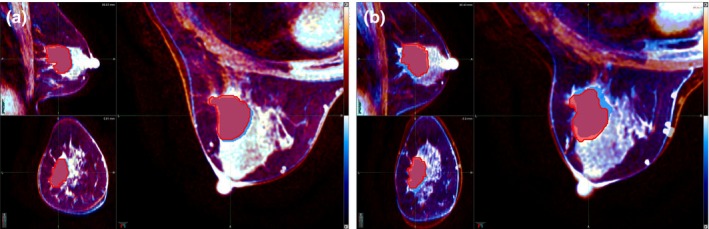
The early and delayed MRI‐images superimposed (a) with the bra and (b) without the bra, for patient ID18. The orange and blue contrast images correspond to the early and delayed images, respectively.
